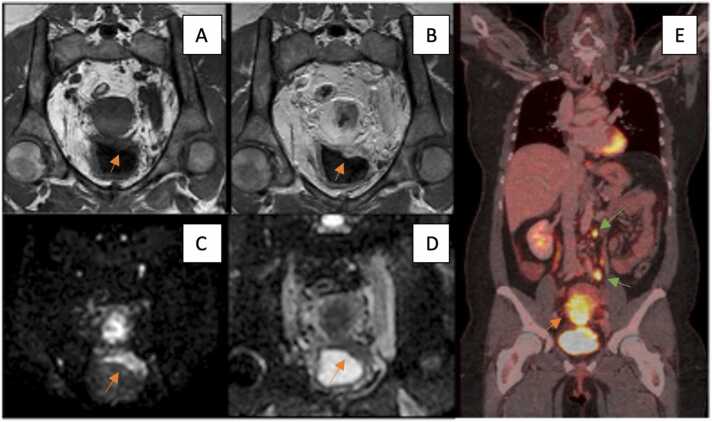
Cervical Carcinoma, MRI and PET/CT imaging. The orange arrow in Picture A (T1W TFE, coronal MRI) shows invasion of the tumour into the posterior wall of the bladder, with the orange arrow in Picture B (Gd T1W TFE, coronal MRI) pointing towards the contrast enhancement of the cervical carcinoma. The arrows in Picture C (ADC, coronal MRI) and D (DWI b=1000, coronal MRI) show restricted diffusion of the cervical carcinoma as well as the posterior wall of the urinary bladder with a low ADC value 0,58 × 10–3 mm2/s of the tumor. Picture E shows a coronal PET/CT with the green arrows pointing towards paraaortic and left iliac lymph node metastases with FDG accumulation. The orange arrow in picture E shows the FDG accumulation of the cervical carcinoma.
